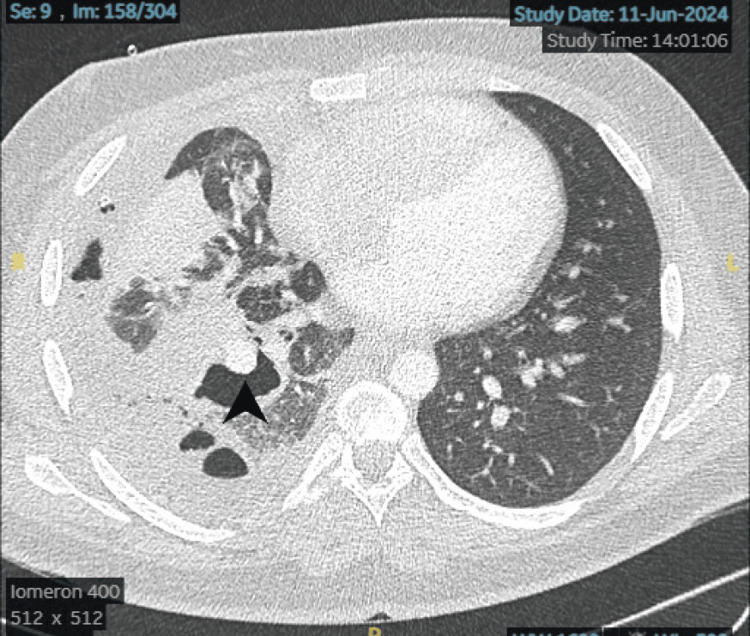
CT aortogram showing a right pulmonary artery aneurysm (arrowhead) located within an infected cavitary lesion in the right lung. The imaging appearance, in the context of active pulmonary tuberculosis, is consistent with a Rasmussen aneurysm. CT: computed tomography
